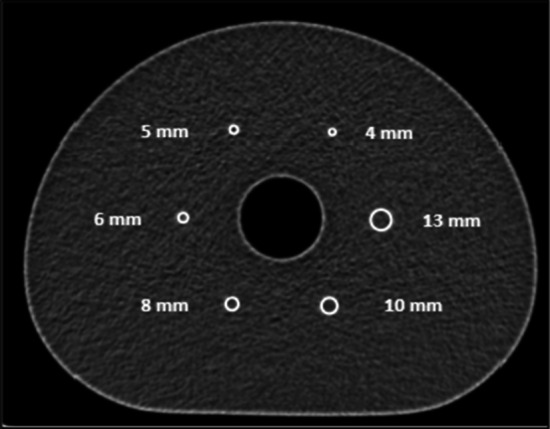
Central section of the spheres from CT images
